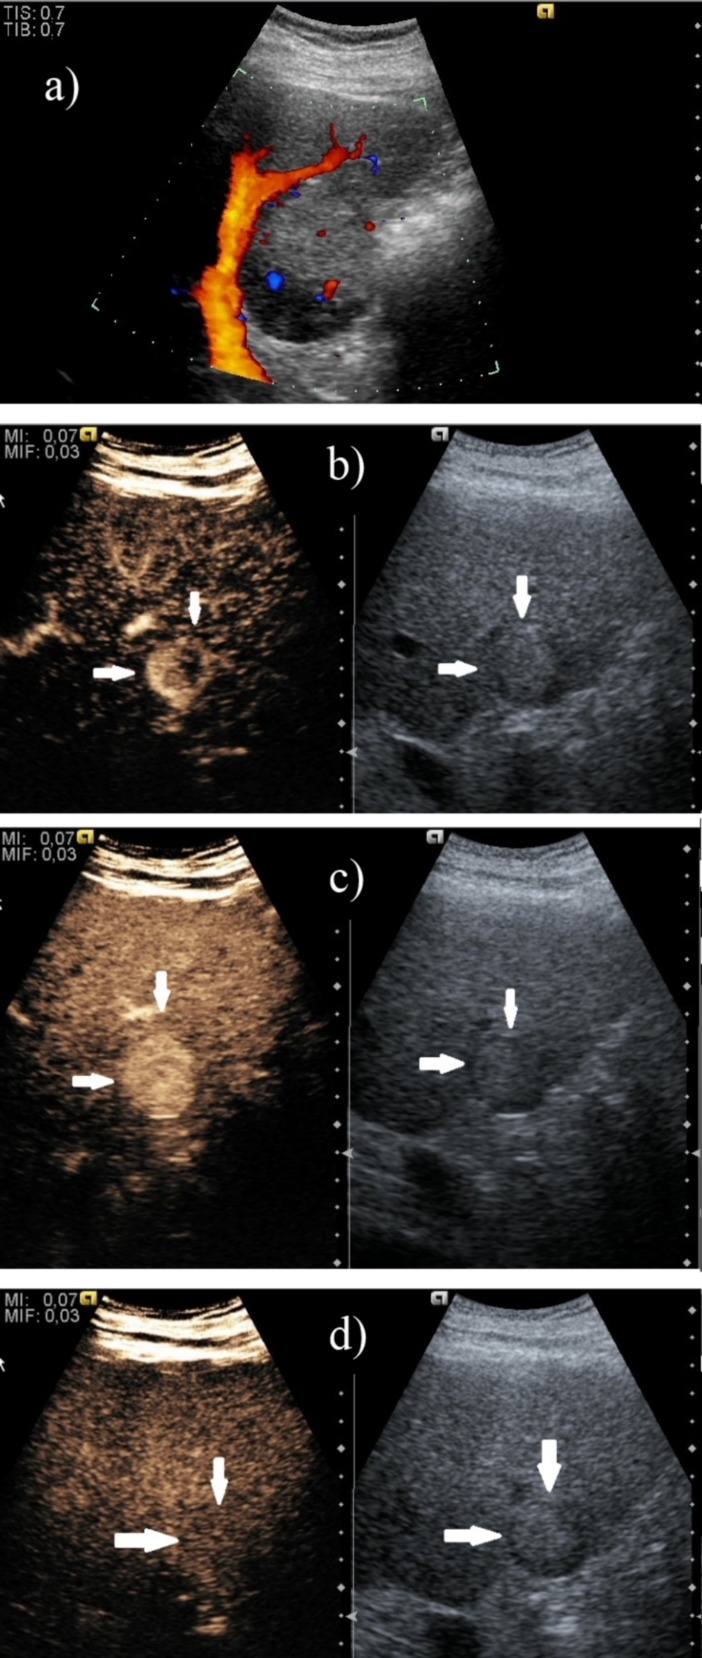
Well-differentiated hepatocellular carcinoma with discreet vascularization on Doppler ultrasound (a), but with intense and heterogeneous hyperenhancement during the arterial phase (b, c) and without washout during the late phase (d)
